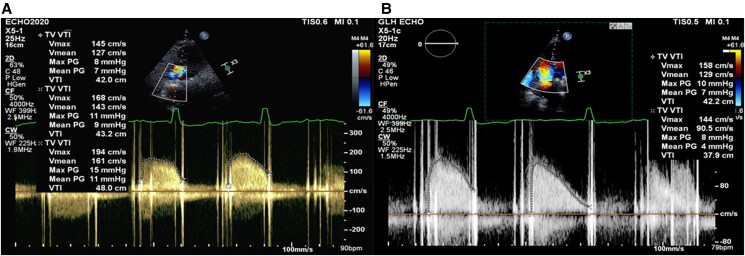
Transvalvular gradient of 27 mm St Jude mechanical tricuspid valve at presentation (*A*) and at 2-month follow-up (*B*). The average transvalvular mean gradient was 8 mmHg in atrial fibrillation with a maximum mean gradient of up to 11 mmHg at presentation. The continuous wave (CW) Doppler exhibits a parabolic profile and long pressure half-time consistent with severe valvular stenosis (*A*). At follow-up, there was a reduction in the average transvalvular mean gradient of 6 mmHg, return of the normal triangular CW profile, and a reduction in pressure half-time (*B*).
